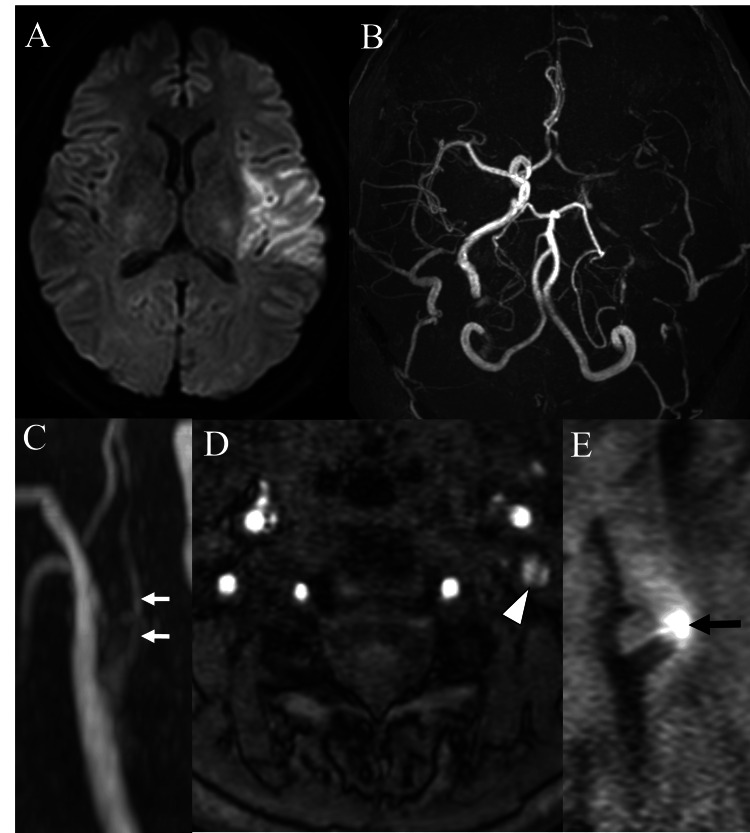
Radiological images at the initial presentation. Diffusion-weighted magnetic resonance (MR) image showing a hyperintense area in the left temporal lobe, indicative of acute infarction (A). Brain MR angiography (MRA) demonstrating reduced signal intensity in the left middle cerebral artery territory (B). Cervical MRA showing near occlusion of the left internal carotid artery (white arrows) with an associated intimal flap (white arrowhead) (C, D). T1-weighted black-blood MRI demonstrating a high signal intensity area in the left ICA, consistent with an intimal hematoma (black arrow) (E). ICA: internal carotid artery; MCA: middle cerebral artery
